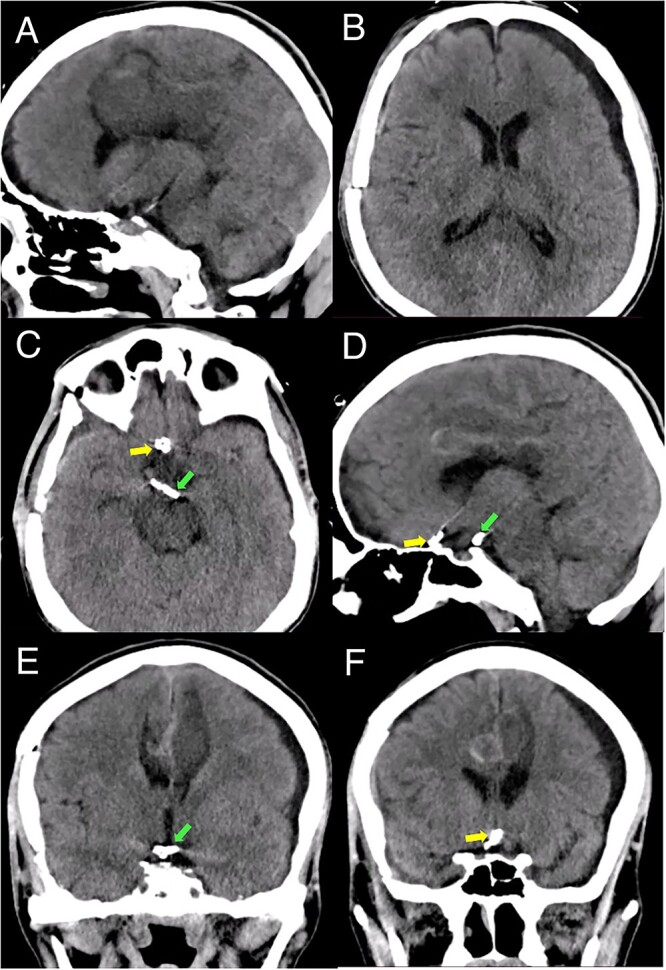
Postoperative head CT. (A, B) Sagittal and axial views show resolution of the hematoma and intraventricular hemorrhage with remaining postinfarction areas. (C–F) Postoperative head CT views depict the placement of clips in the accessory A2 artery (yellow arrow) and basilar aneurysm (green arrow).
